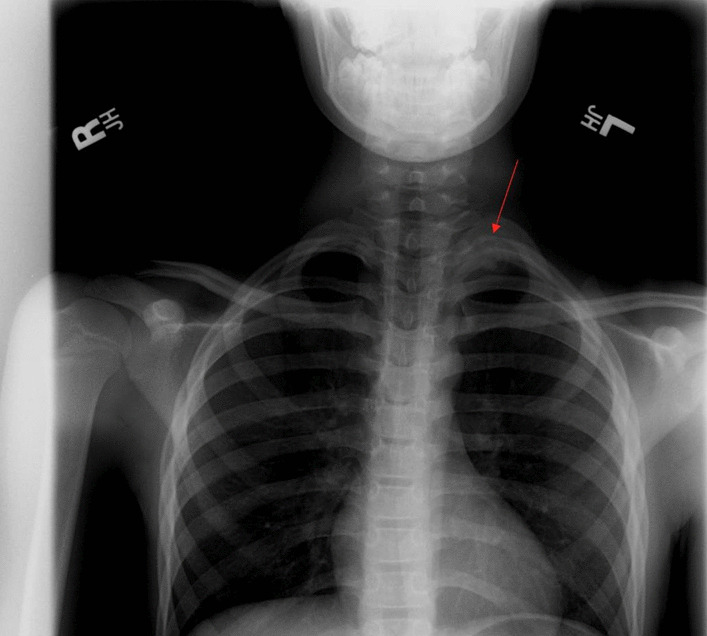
Initial chest X-ray with evidence of left first rib pseudoarthrosis (red arrow is pointing to the pseudoarthrosis present in the patient’s first rib)
